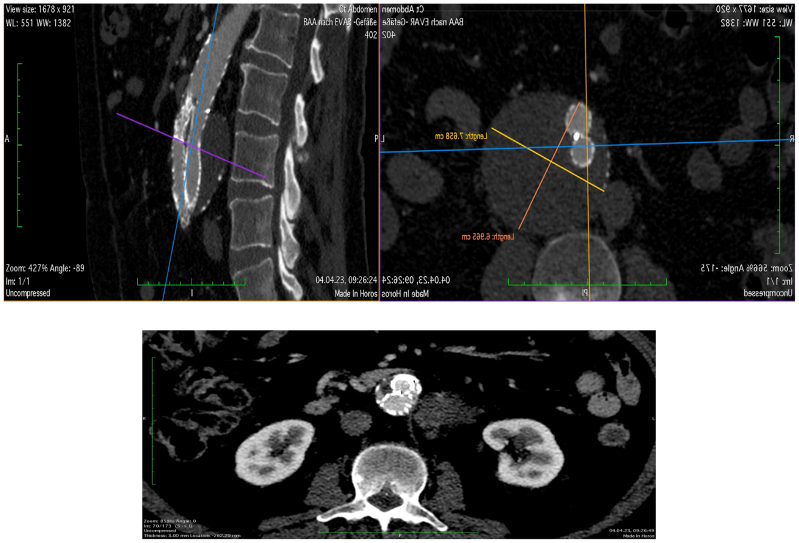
Computed tomography (CT) scan of patient 2.
